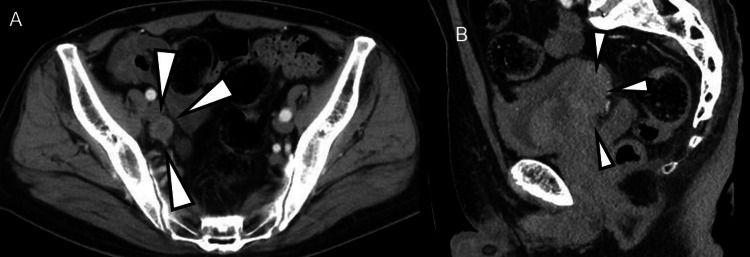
Bladder cancer and lymph node involvement at the time of the initial diagnosis (A) Computed tomography revealed left hydronephrosis, a bladder tumor on the right lateral wall, and right external iliac LN involvement at the time of the initial diagnosis. (B) T2-weighted magnetic resonance imaging prior to our therapy revealed a bladder tumor with invasion of the surrounding fibroadipose tissue at the right lateral wall before chemotherapy.
